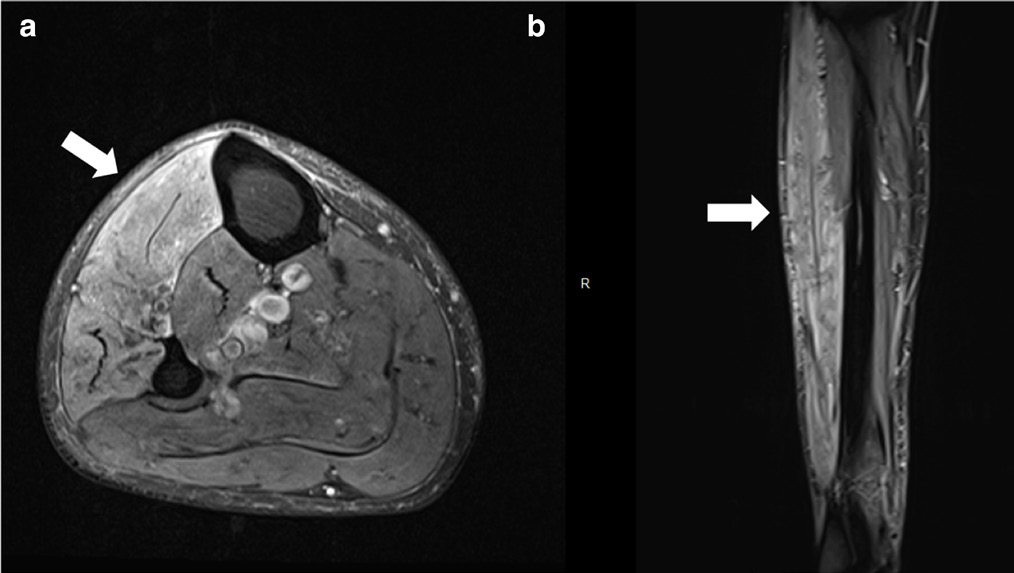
Leg MR imaging demonstrated (A) axial and (B) coronal T2 fat-saturated diffuse muscle edema, involving the anterolateral muscular compartment of the leg.
